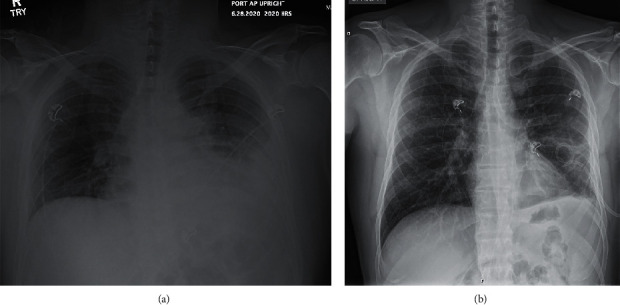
Xray before and after pigtail chest tube placement on second hospitalization.
